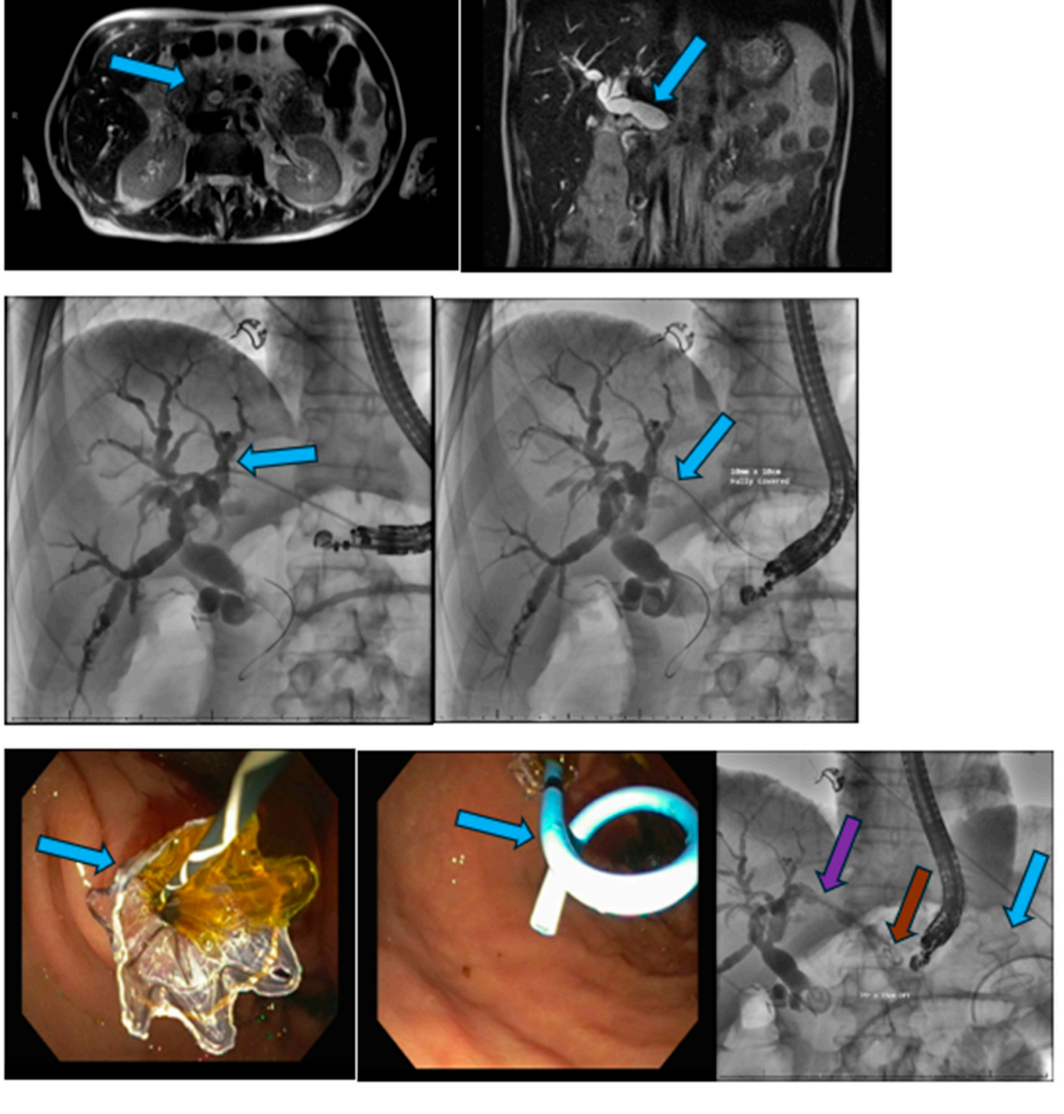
An axial view of a magnetic resonance cholangiopancreatography image (top left image) reveals a 2.1 cm pancreatic head mass (blue arrow) and marked intrahepatic and extrahepatic biliary dilation (top right image) with the common bile duct being approximately 1.98 cm in diameter (blue arrow). Fluoroscopy image (middle left) reveals puncture from the stomach to the left intrahepatic duct using a 19-gauge needle, a guidewire in place and an intraoperative cholangiogram with significant intrahepatic and extrahepatic biliary dilation. Fluoroscopy image (middle right, arrow) and endoscopy image (bottom left, arrow) reveals a 10 mm × 10 cm fully covered, self-expandable metal stent, which was inserted into the biliary tree to create an endoscopic ultrasound-guided hepaticogastrostomy. Subsequently, a 7-French × 15 mm double-pigtail stent was inserted in the self-expandable metal stent (bottom middle image, arrow) for biliary drainage. The bottom right image reveals the self-expandable biliary stent (purple arrow) and the double-pigtail stent (brown arrow). Additionally, a lumen-apposing metal stent was used to perform a gastroenterostomy due to gastric outlet obstruction (blue arrow). Image courtesy of Amar Vedamurthy, Division of Gastroenterology and Hepatology, Virginia Mason Franciscan Health, Seattle, WA, USA.
